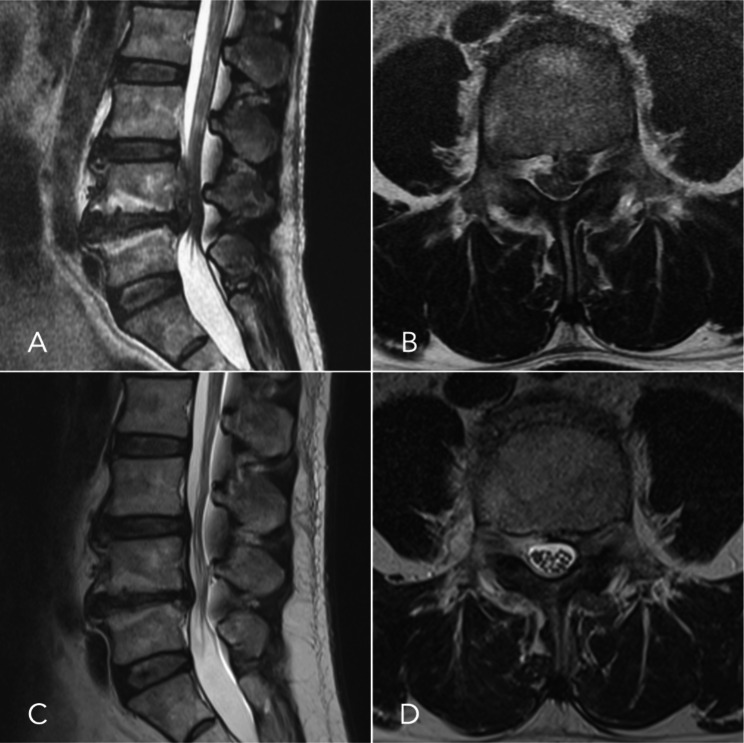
(A, B) Pre-operative MRI revealed a highly upward-migrated disc herniation extending beyond zone 1 with thecal sac compression at L4–5 level. (C, D) Post-operative MRI showed that the ruptured disc was removed entirely with re-expansion of the thecal sac and no soft tissue injury
